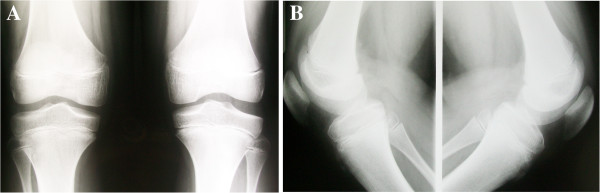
Antero-posterior (A) and lateral (B) standing X-rays showing bilateral squaring of the lateral femoral condyle and hypoplasia of the lateral tibial spines.
